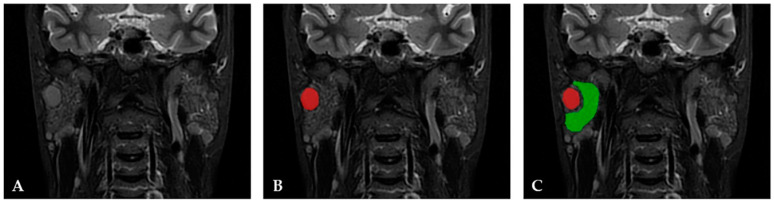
(A) MRI coronal STIR PROPELLER sequence of a 45-year-old female patient diagnosed with primary Sjögren’s syndrome and non-Hodgkin lymphoma, MALT subtype in the right parotid gland. (B) The software automatically traced the region of interest (ROI) covering the focal lesion corresponding to lymphoma using geometric and gradient coordinates (red area). (C) A second ROI was manually delineated (green area), covering the parotid gland’s parenchyma surrounding the focal lesion (this 2D segmentation was used for the textural analysis).
